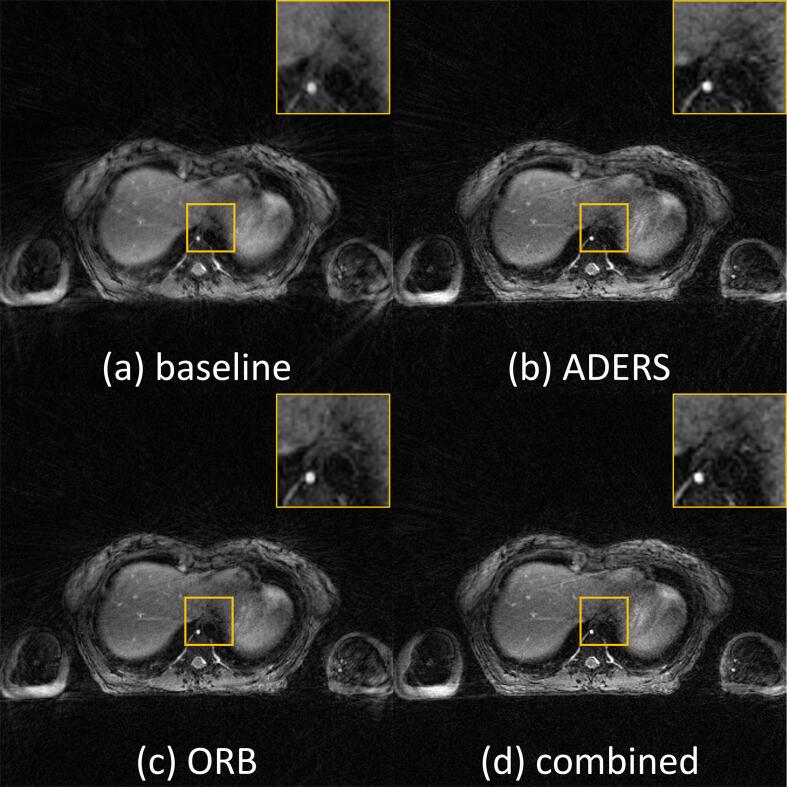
End-inspiratory phase of 4D-MRI acquired with abdominal compression and reconstructed from the same acquisition using the different data preparation strategies. Details from fine non-moving structures appear sharper in (b), (c) and (d) compared with the baseline (a).
